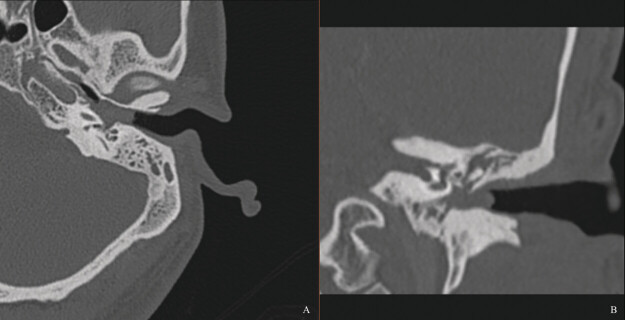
CT of the left temporal bone showing middle ear opacification and complete mastoid opacification with new bone formation around the oval window. (
